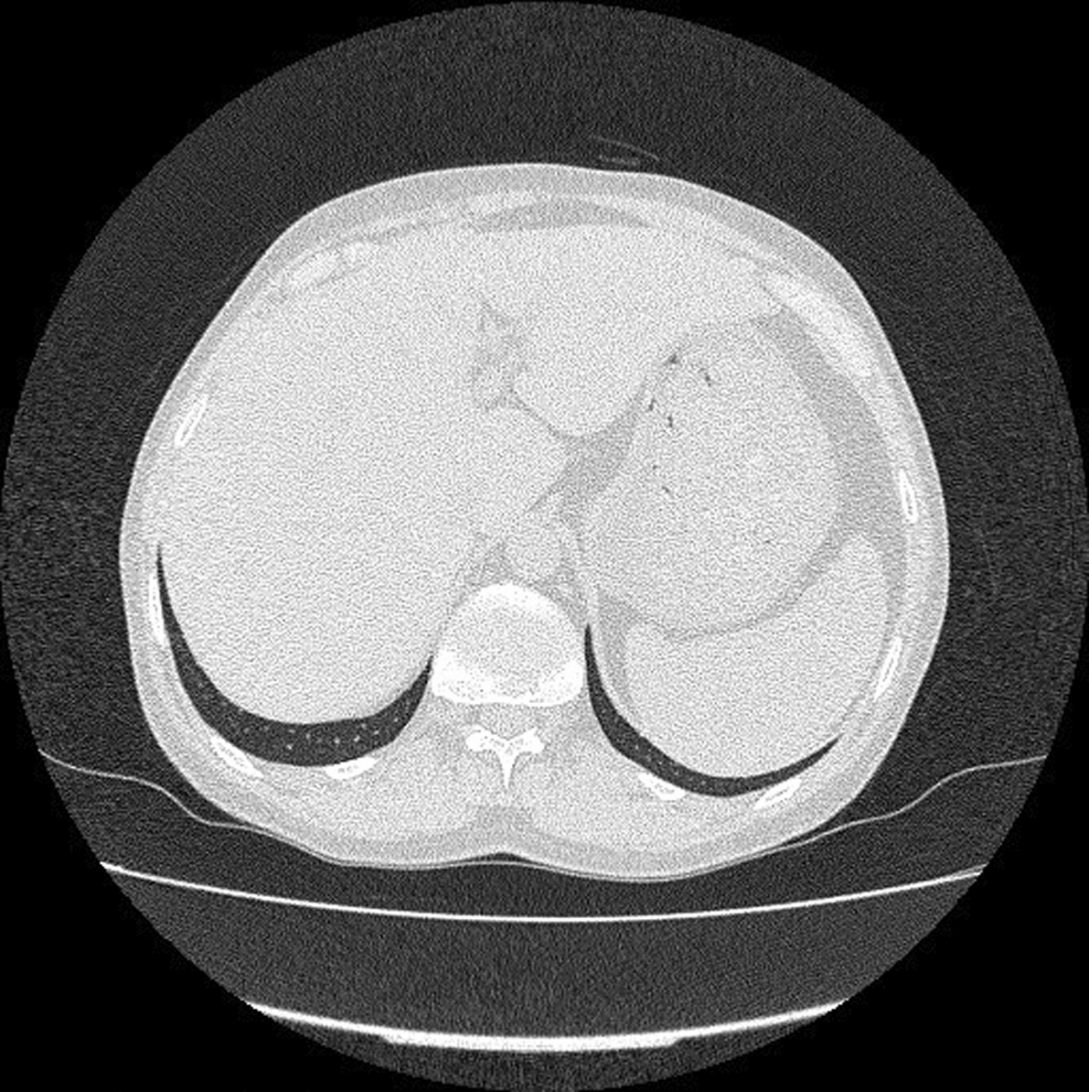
Abdominopelvic computed tomography (CT) scan confirmed hepatosplenomegaly.
